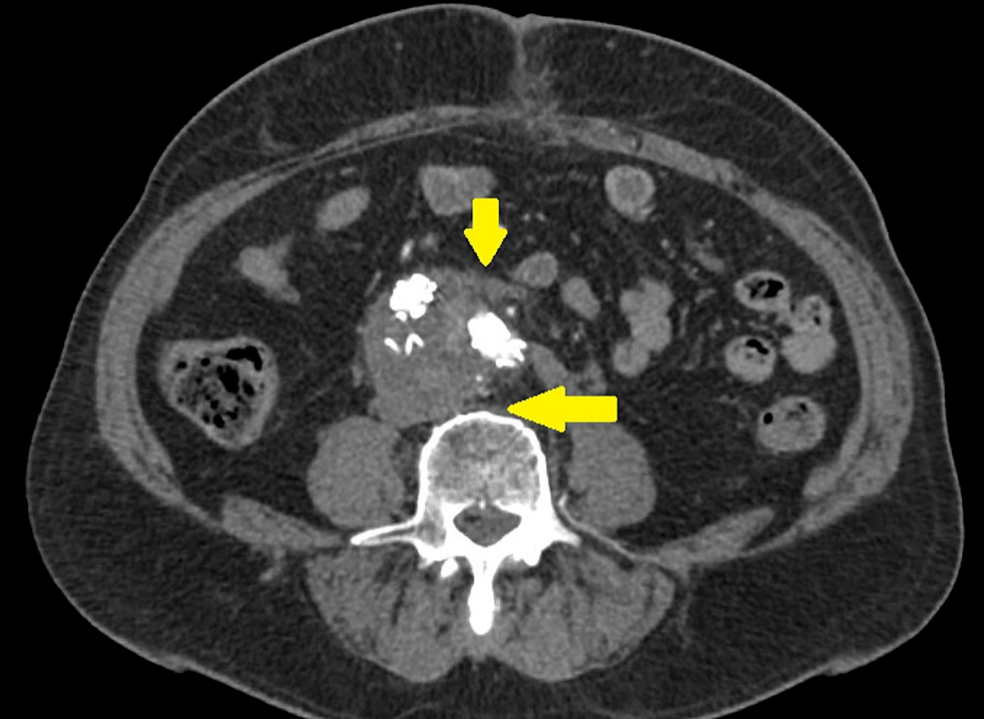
Follow-up computed tomography angiography shows graft salvage and no evidence of endoleak type II.
